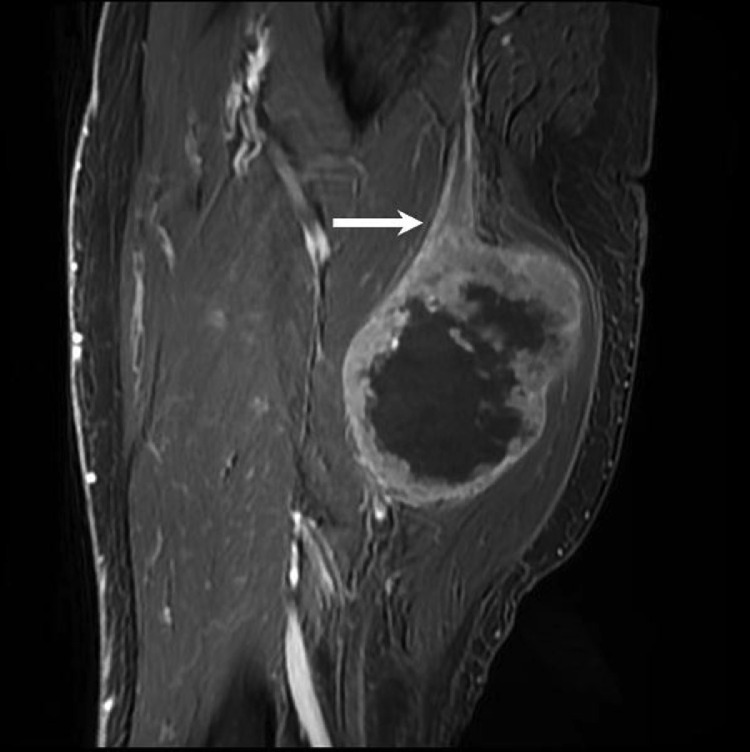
Sagittal T1 fat-saturated MRI image with contrast shows predominantly peripheral enhancement of the mass with extensive central necrosis. There is abnormal enhancement of the sciatic nerve which leads into the mass (arrow).
